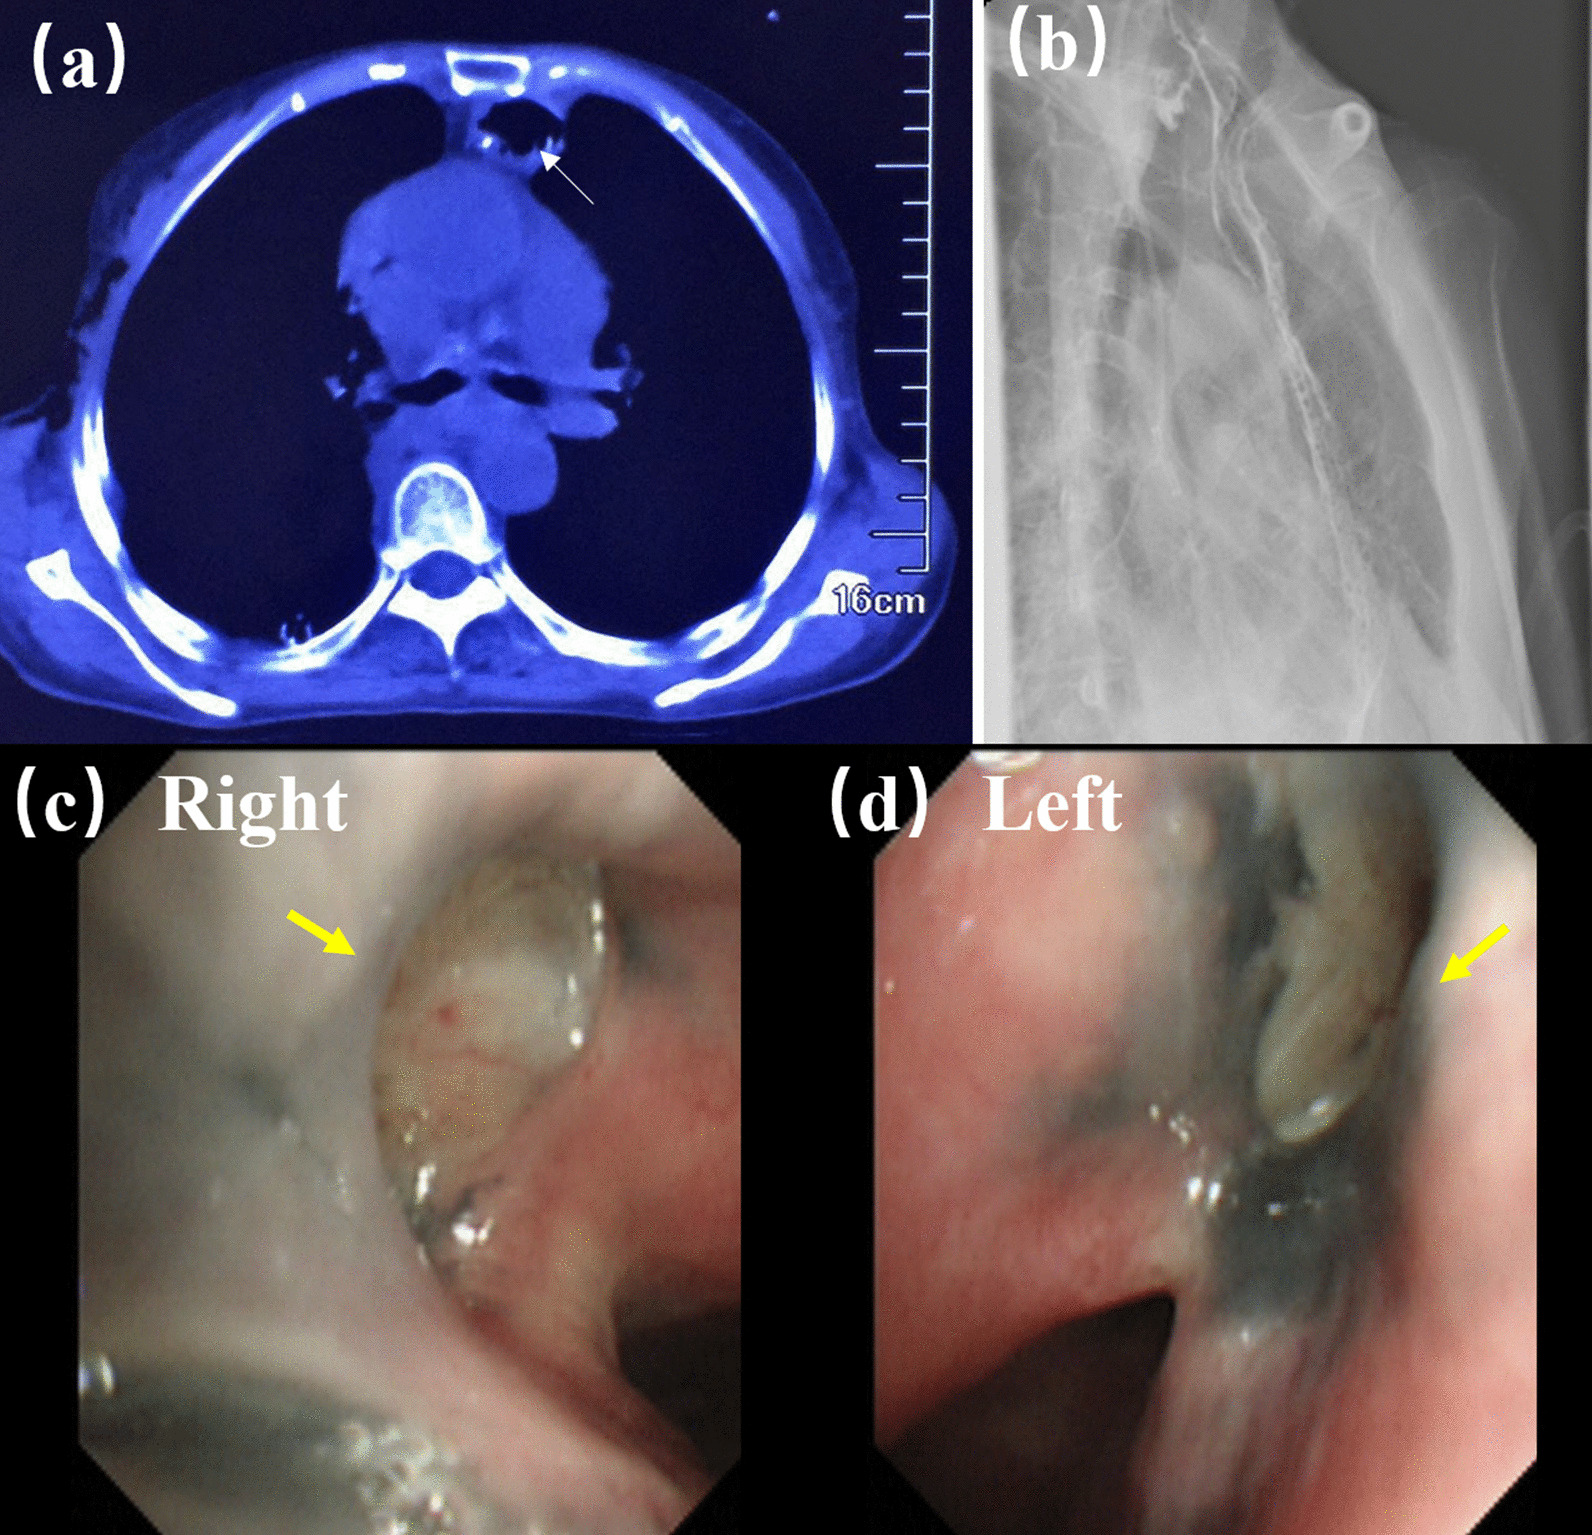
Postoperative imaging examination (yellow arrows indicate closured fistula). a CT results showing complete disappearance of the fistula (the white arrow points to the tubular stomach). b Barium esophagogram showing the absence of anastomotic leakage. c, d Bronchoscopy showing that the fistula was repaired well
